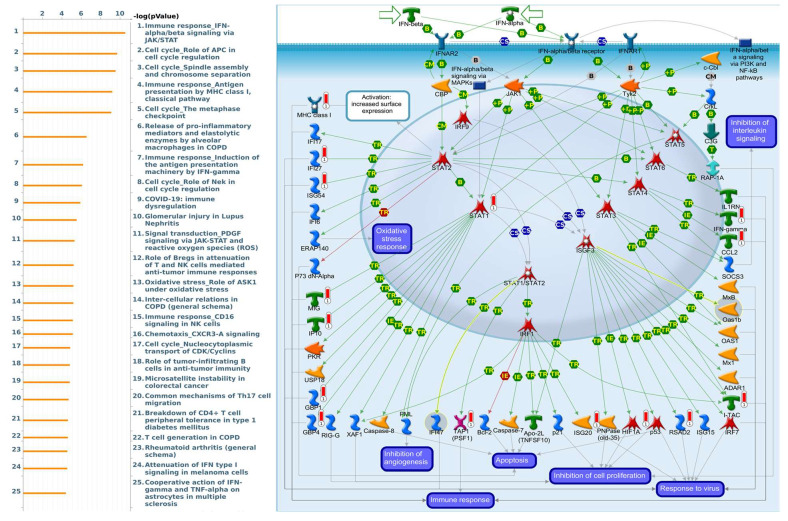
MetaCore pathway analysis of the coexpression gene network of proteasome 20S subunit alpha 4 (PSMA4) in breast cancer patients. We used the MetaCore platform to analyze genes coexpressed with PSMA4 from the associated METABRIC and TCGA datasets, and downstream pathway analyses revealed that “Immune response_IFN-alpha and beta signaling via JAK/STAT” participates in breast cancer development.
